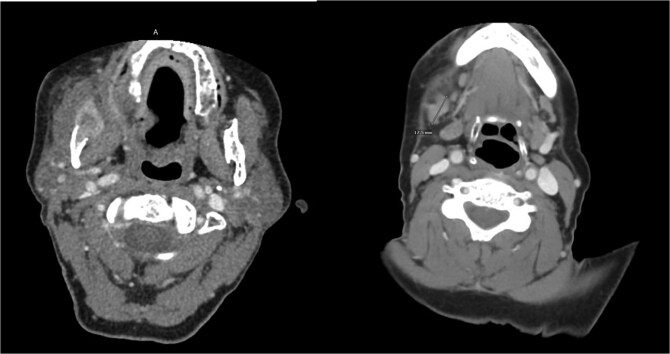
CT neck with contrast, near time of initial diagnosis: Infiltrative, erosive 3.8 × 2.5 × 4.1 cm soft tissue mass is seen involving the right hemimandible with locoregional soft tissue infiltration. Enlarged right level 1B lymph nodes are seen measuring up to 1.1 × 1.6 cm which may be reactive in nature or reflect nodal metastases.
